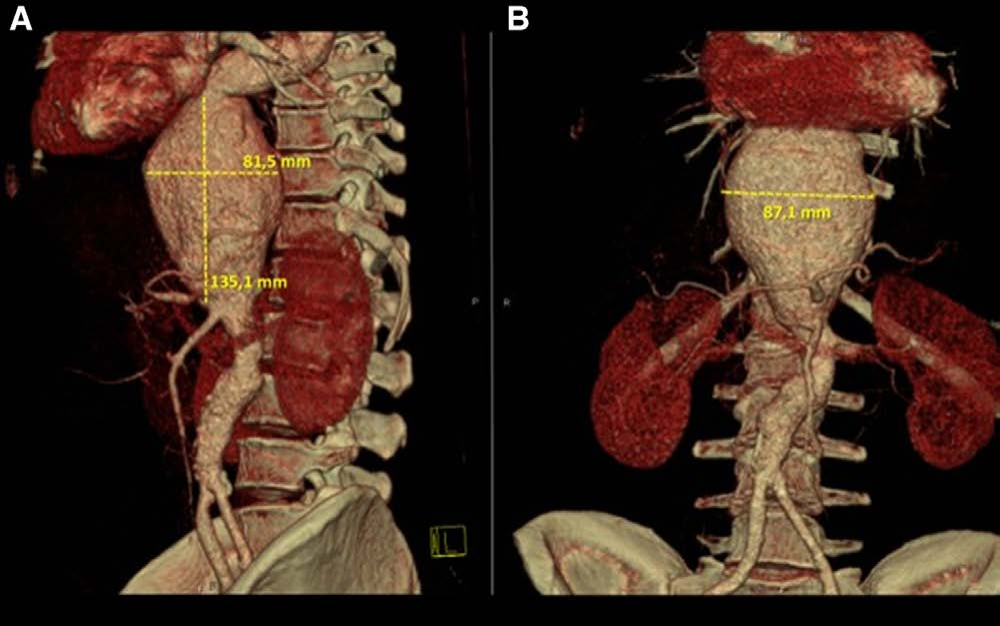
VRT of CT angiography. VRT from CT angiography showing a thoracoabdominal aortic aneurysm. Left lateral view of the thoracoabdominal aorta with evident fusiform aneurysmal dilation (A). Anterior view of the thoracoabdominal aorta demonstrating the full craniocaudal extent of the aneurysm (B). CT = computed tomography, VRT = volume-rendered 3D reconstruction.
